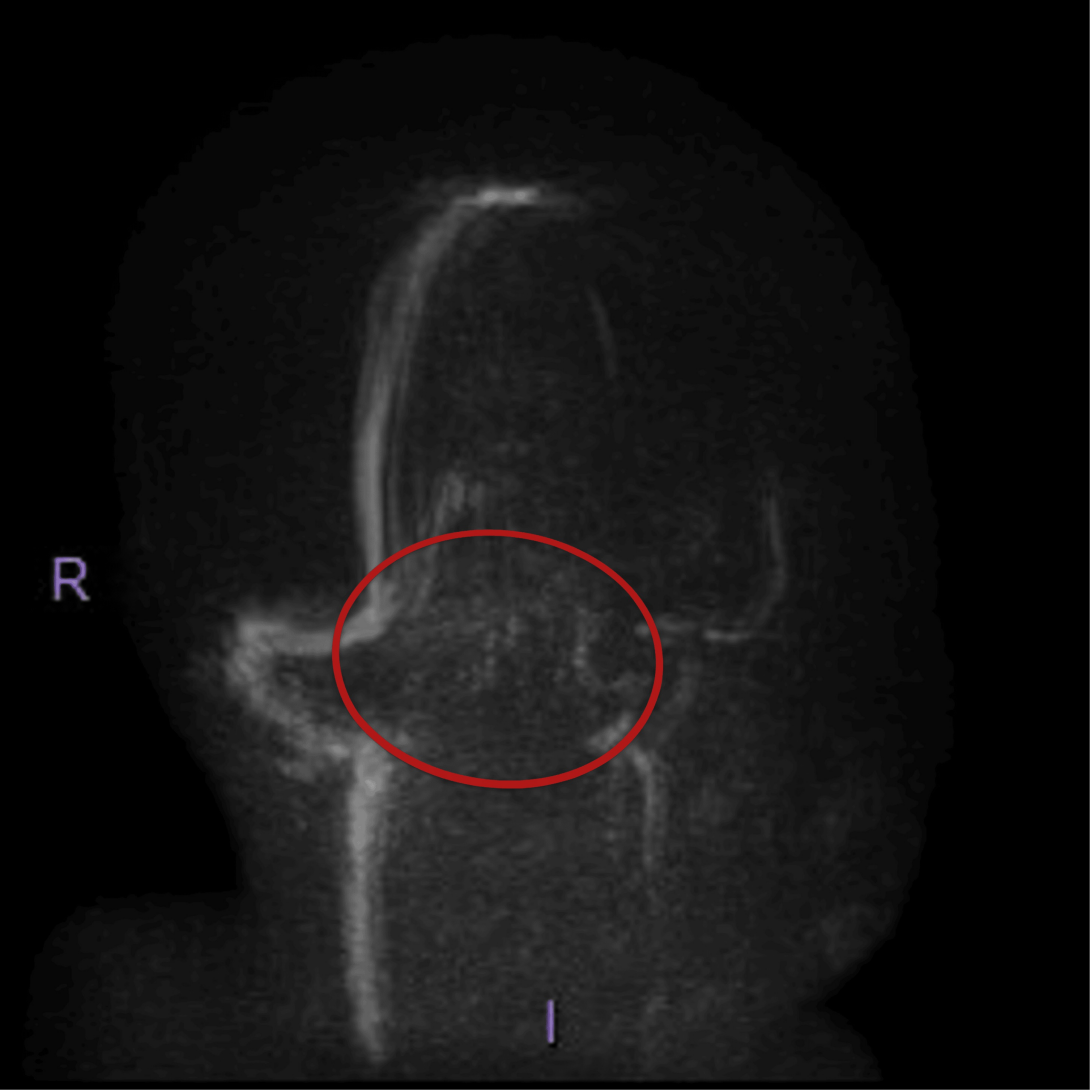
MRI venogram showing signal loss in the left sigmoid and transverse sinuses (red circle)
